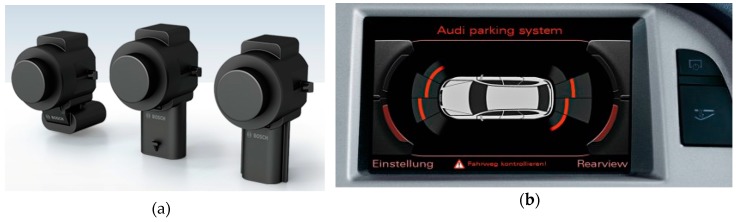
(a) Ultrasonic car sensors from Bosch; (b) an assistance parking system from Audi.
